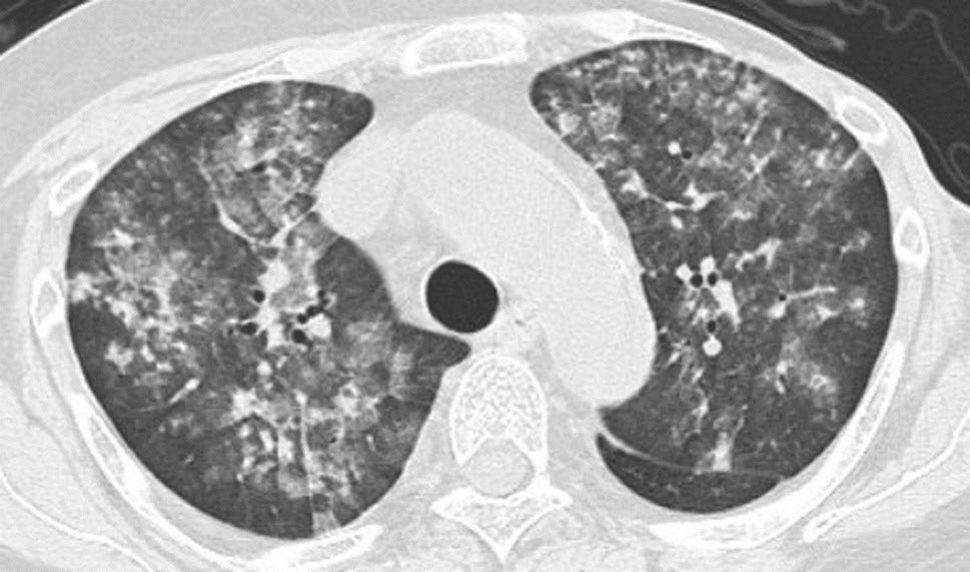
A 56-year-old woman with diffuse alveolar hemorrhage under treatment for thrombocytopenia and uterine cancer. High-resolution computed tomography shows extensive ground-glass attenuation with multiple nodular lesions
